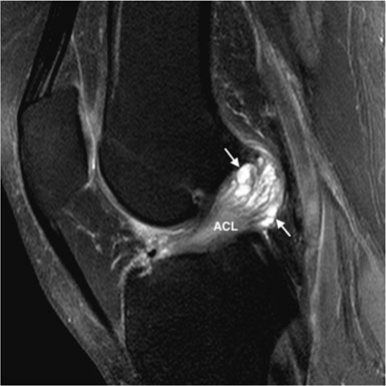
Anterior cruciate ligament ganglion cyst incidentally found in a 58-year-old woman during an MRI scan performed in the setting of a knee sprain. Sagittal FS PD-WI shows an enlarged anterior cruciate ligament due to a multiloculated cystic lesion (arrows) embedded within its fibers. ACL, anterior cruciate ligament
